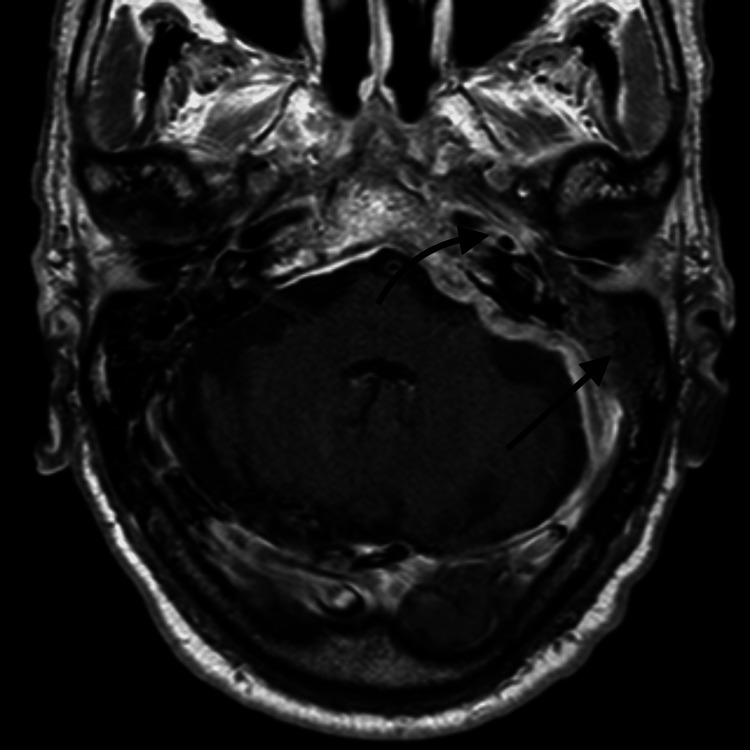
T1-weighted MRI with gadolinium documenting septic left lateral sinus thrombosis with apical petrositis.
